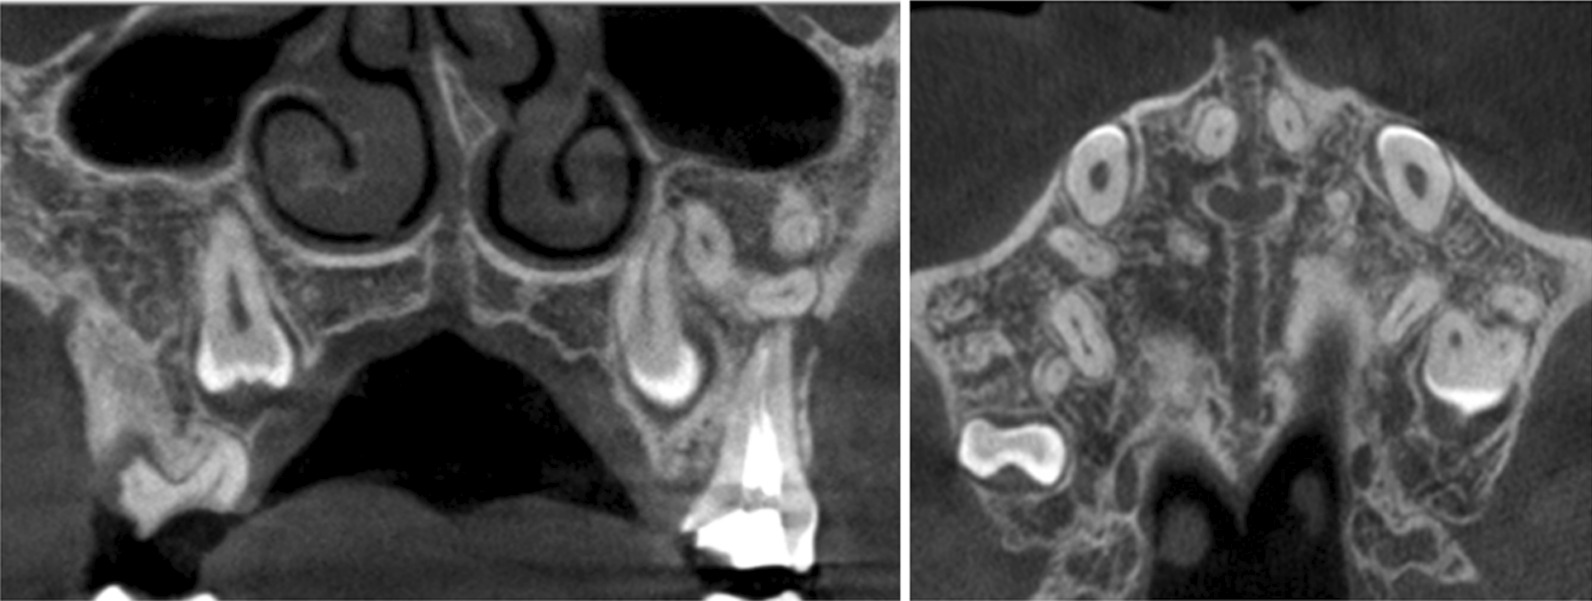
CBCT images 1 month after MARPE
